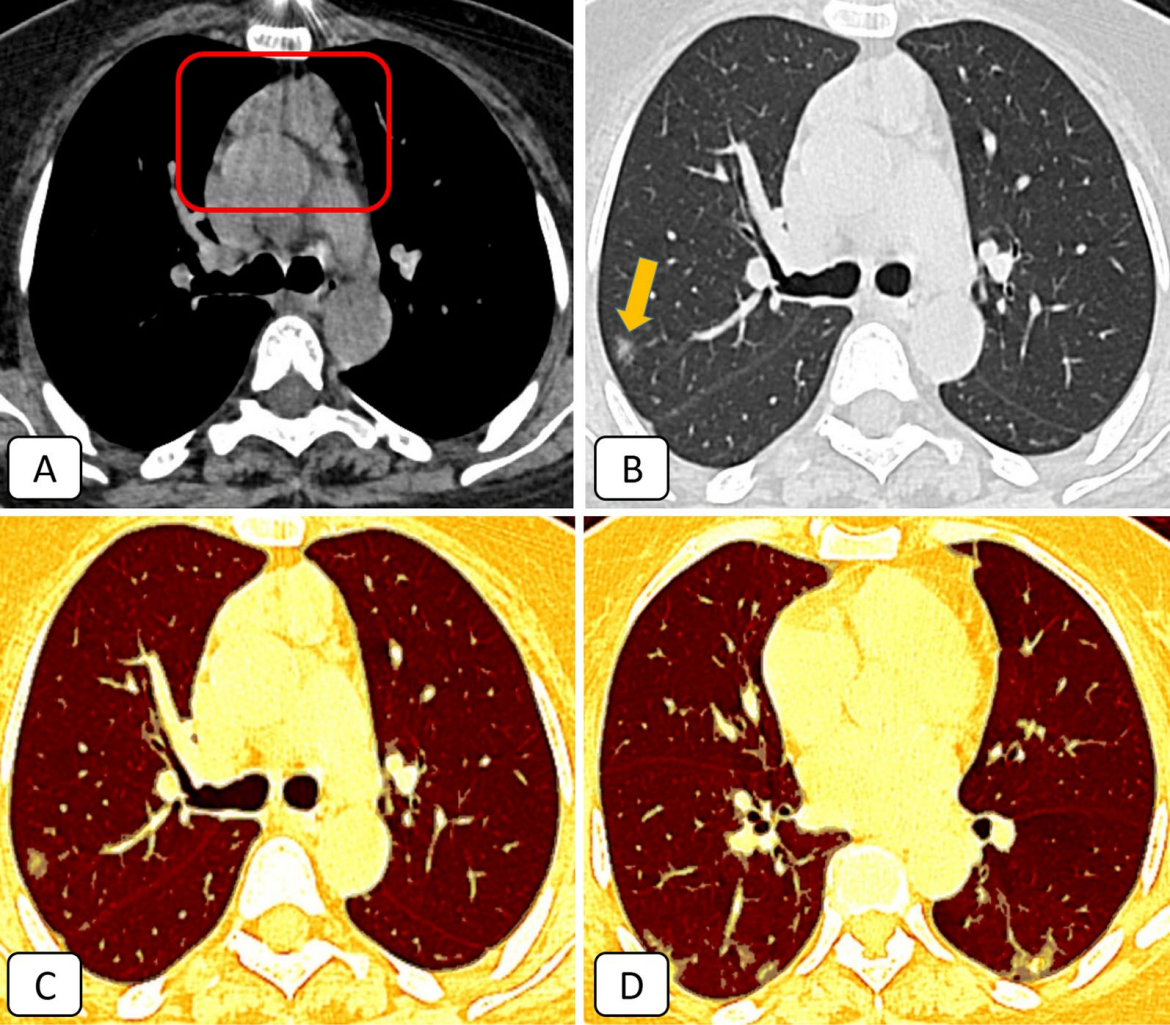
A 57-year-old female COVID-19 patient complained of loss of taste and smell: A Axial mediastinal window CT showing solid soft tissue replacement of the thymic gland (grade 0) [red square]. B Axial lung window CT showed sub-pleural small ground-glass nodular opacity in the posterior segment of the right upper lobe (orange arrow). C–D Axial 3D volumetry cuts highlighted the bilateral basal small sub-pleural ground-glass opacities. The pathological lung volume was around 1% of the total lung volume
